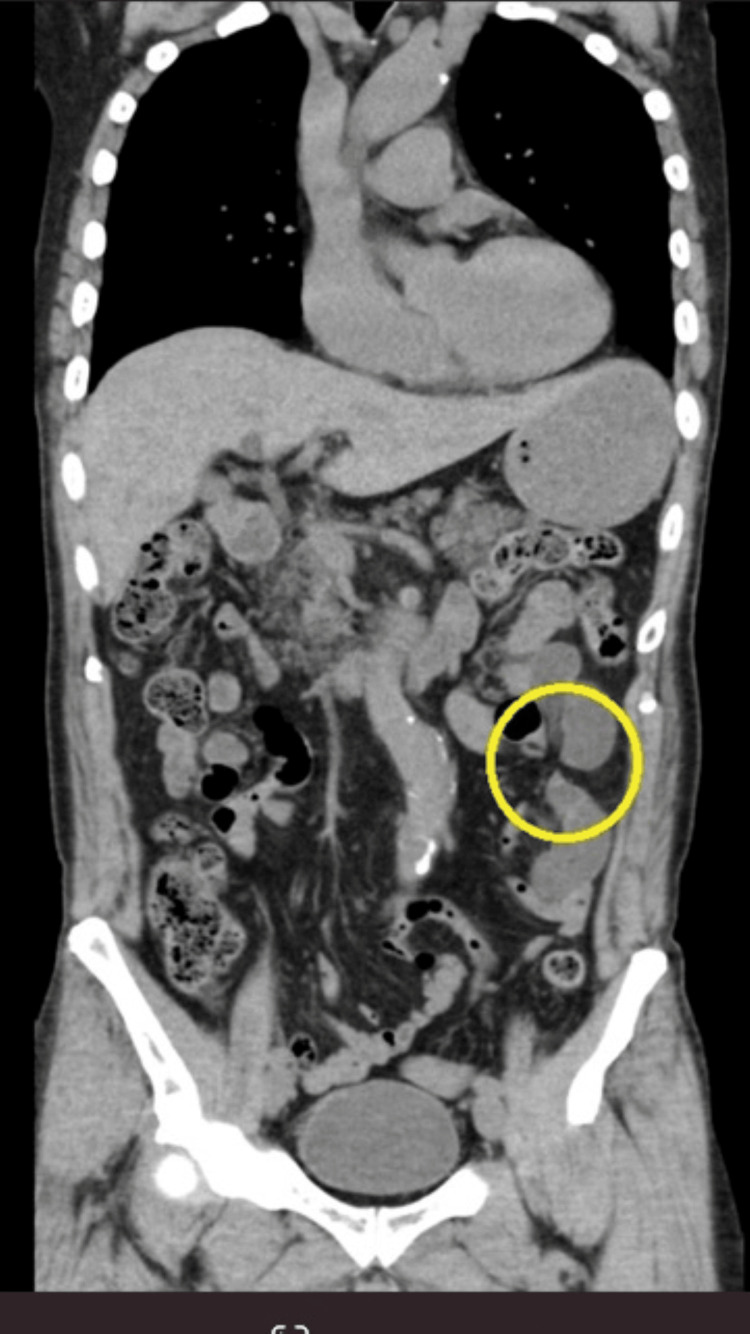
Strangulated ileus (closed loop) seen on plain CT The patient was administered intravenous acetaminophen for pain management, and non-contrast CT imaging suggested a strangulated ileus (the yellow circle).
